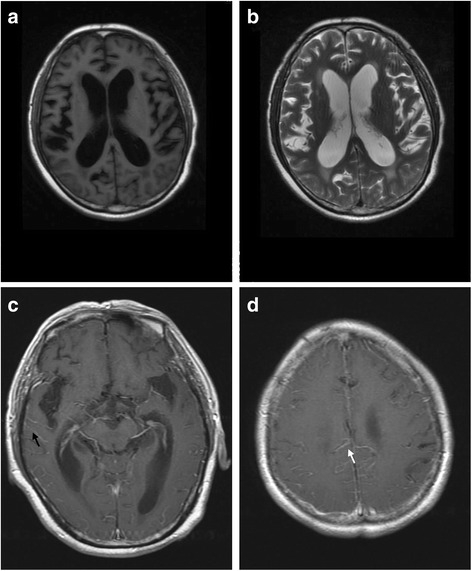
Head MRI of the patient. a, b T1- and T2-weighted images indicate supratentorial ventricular enlargement and abnormal signals in periventricular white matter, respectively. c, d Head MRI with gadolinium enhancement indicated leptomeningeal enhancement mainly located in the right temporal lobe (black arrow) and posterior cingulate gyri (white arrow)
